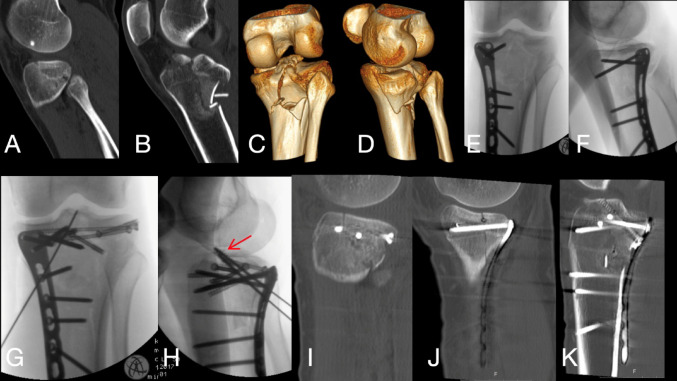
Computed tomography images in sagittal views A, B and three-dimensional reconstructions C, D demonstrate a tibial plateau fracture consistent with a flexion-type injury mechanism. The surgical procedure was completely perfomed in prone position. The operation was started by a posteromedial approach with a reduction and fixation of the main posteromedial fragment by buttress plate osteosynthesis (E, F). The bony avulsion of the PCL was reduced and fixated by two screws (G). The bony ACL avulsion was reduced by an ACL drill guide, which was introduced from posterior between ACL and PCL. The fragment was reduced with the drill guide, which had a sharp tip to reduce the fragment. Two guide wires from posterior were then drilled retrograde in the fragment (H). Two cannulated screws were inserted retrograde to fix the fragment (H, red arrow). The posterolateral fragment was reduced by using the posteromedial approach. After elevation of the posterolateral fragment with a chisel, the articular surface was supported by an allograft bone block (I). Fixation was performed with 3 cannulated, percutaneous screws in #-technique. Postop CT scan reveals an anatomical reduction of the articular surface with a proper fixation of the fracture (I–K)
